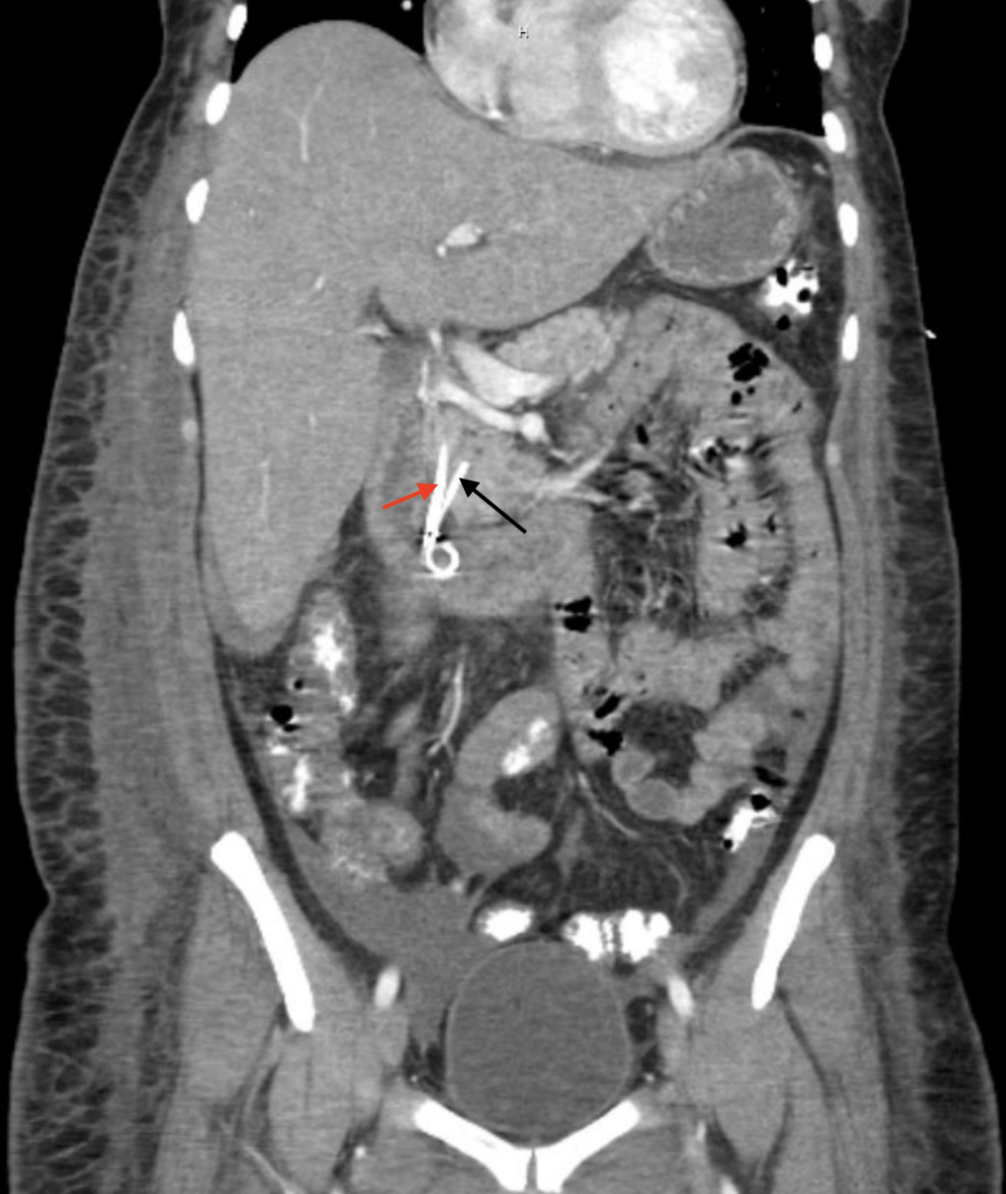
CT Abdomen & Pelvis with Contrast - Common Bile Duct & Pancreatic Duct Stents Black arrow: 4-French 3-cm pancreatic duct stent. Red arrow: 8.5-French 9-cm common bile duct stent.
